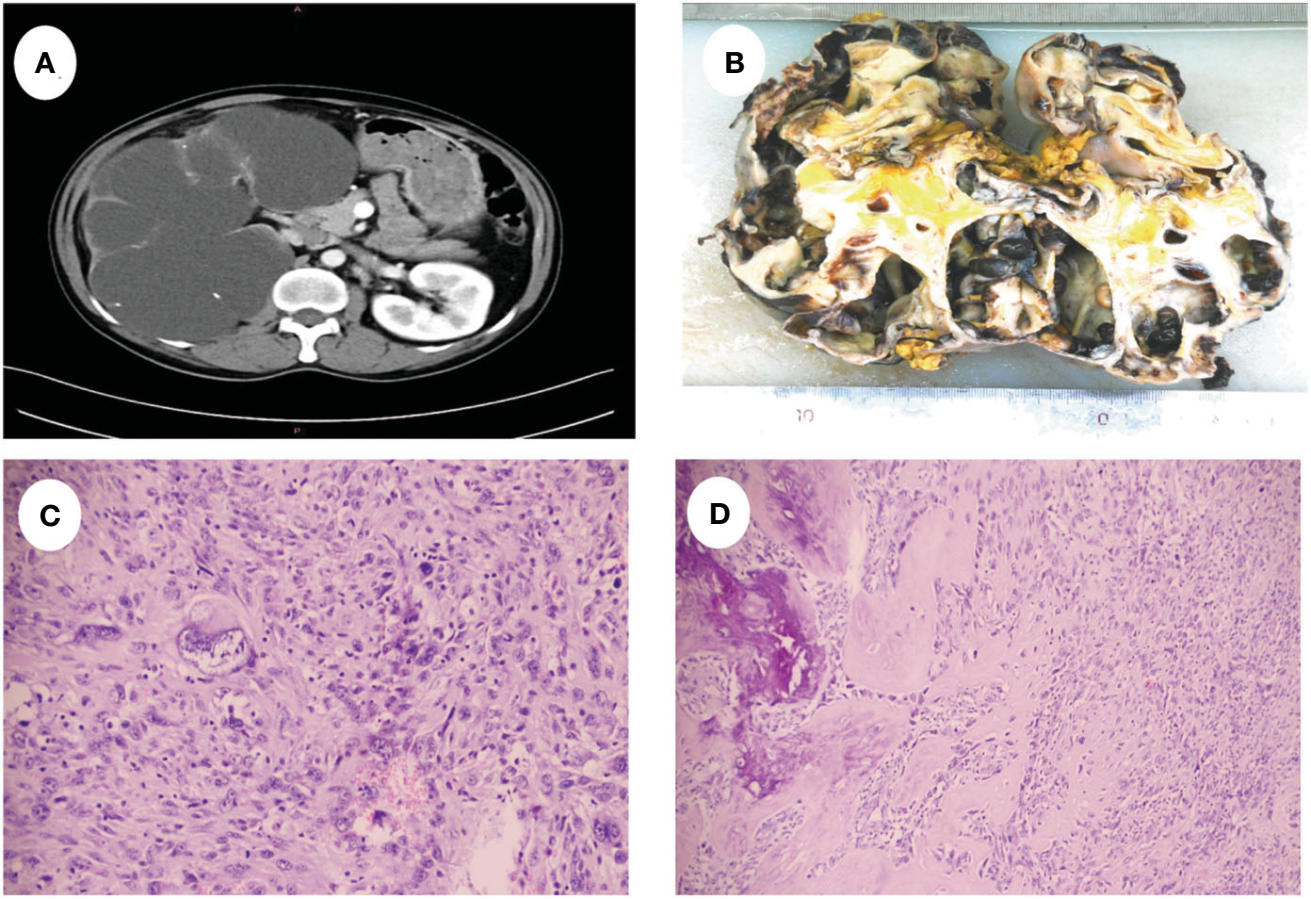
(A) CT shows severe hydronephrosis of the right kidney. (B) Shows the gross appearance of the right kidney, the size was about 20cm x 15cm. (C) The tumor cells were disorganized and showed sarcomatoid changes with marked heterogeneity. (D) Tumor cells are spindle-shaped with ossification formation.
